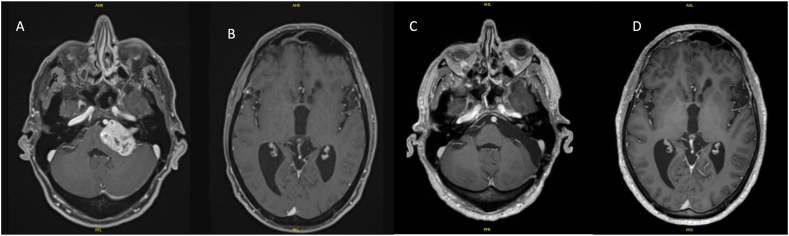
(A) Axial T1-weighted MRI showing a 3,5 cm left CPA tumor with (B) hydrocephalus. (C) A postoperative T1-weighted MRI with gadolinium confirmed total removal of tumor and (D) regression of hydrocephalus one year later.
